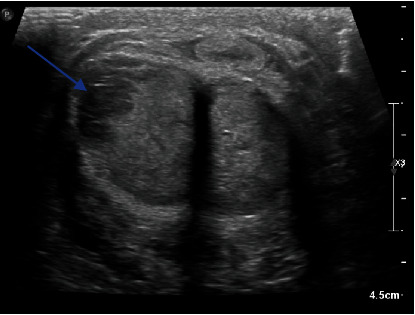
Penile ultrasound axial cuts showing tumor invasion of the corpora cavernosa on the right (blue arrow).
